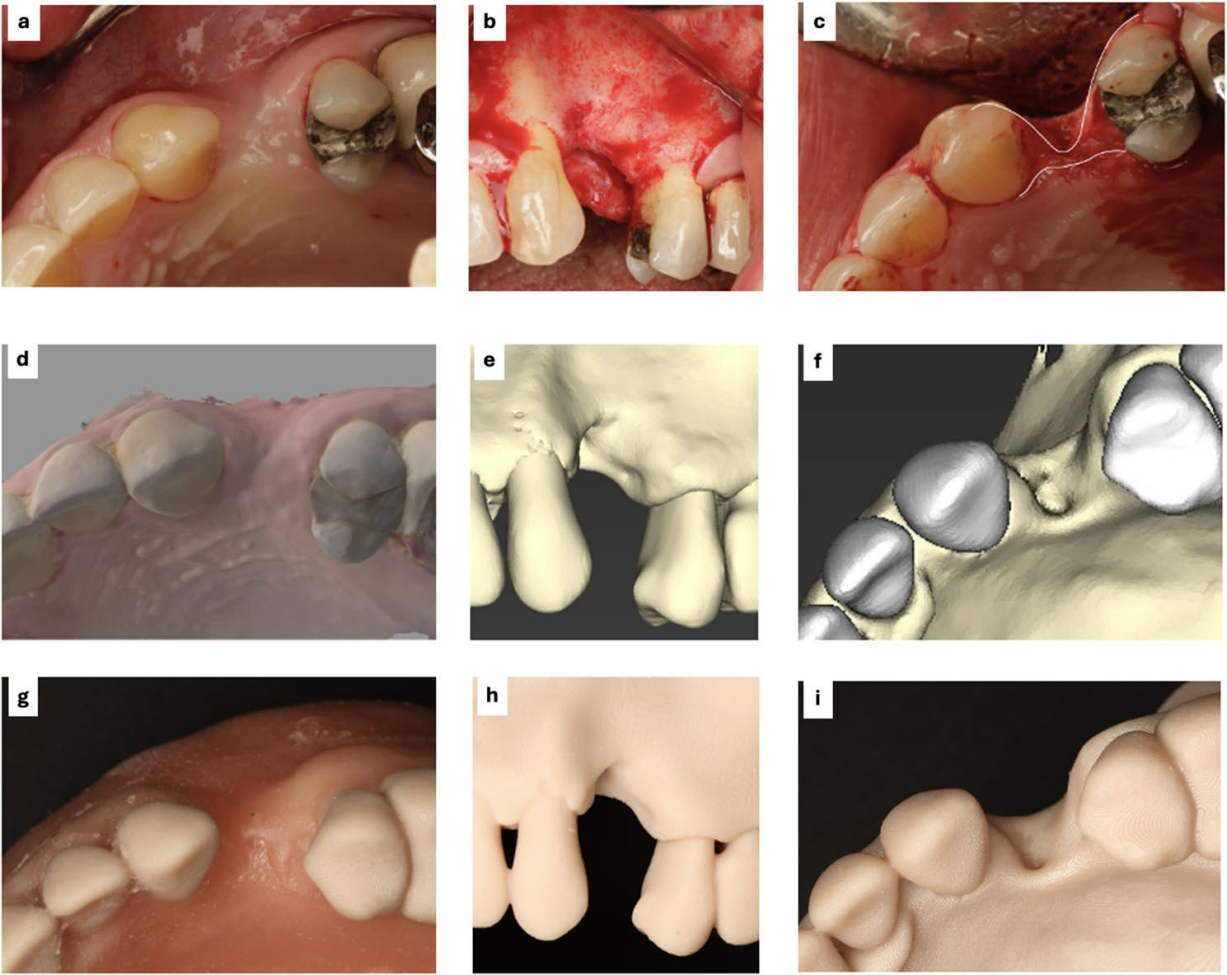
(a) Patient’s intraoral occlusal view photograph of the #24 defect site, b Buccal view of the site alveolar bone after flap elevation during surgery, c Occlusal view of the alveolar bone during surgery, d Intraoral scan showing the occlusal view of the #24 defect site, e and (f) Buccal and occlusal views of the defect site on CBCT images, respectively, g Final 3D-printed model showing the occlusal view of the #24 defect site, h and (i) Buccal and occlusal views of the 3D-printed bone model, respectively. Patient intraoral photos are courtesy of Dr. Sepehr Torabi
